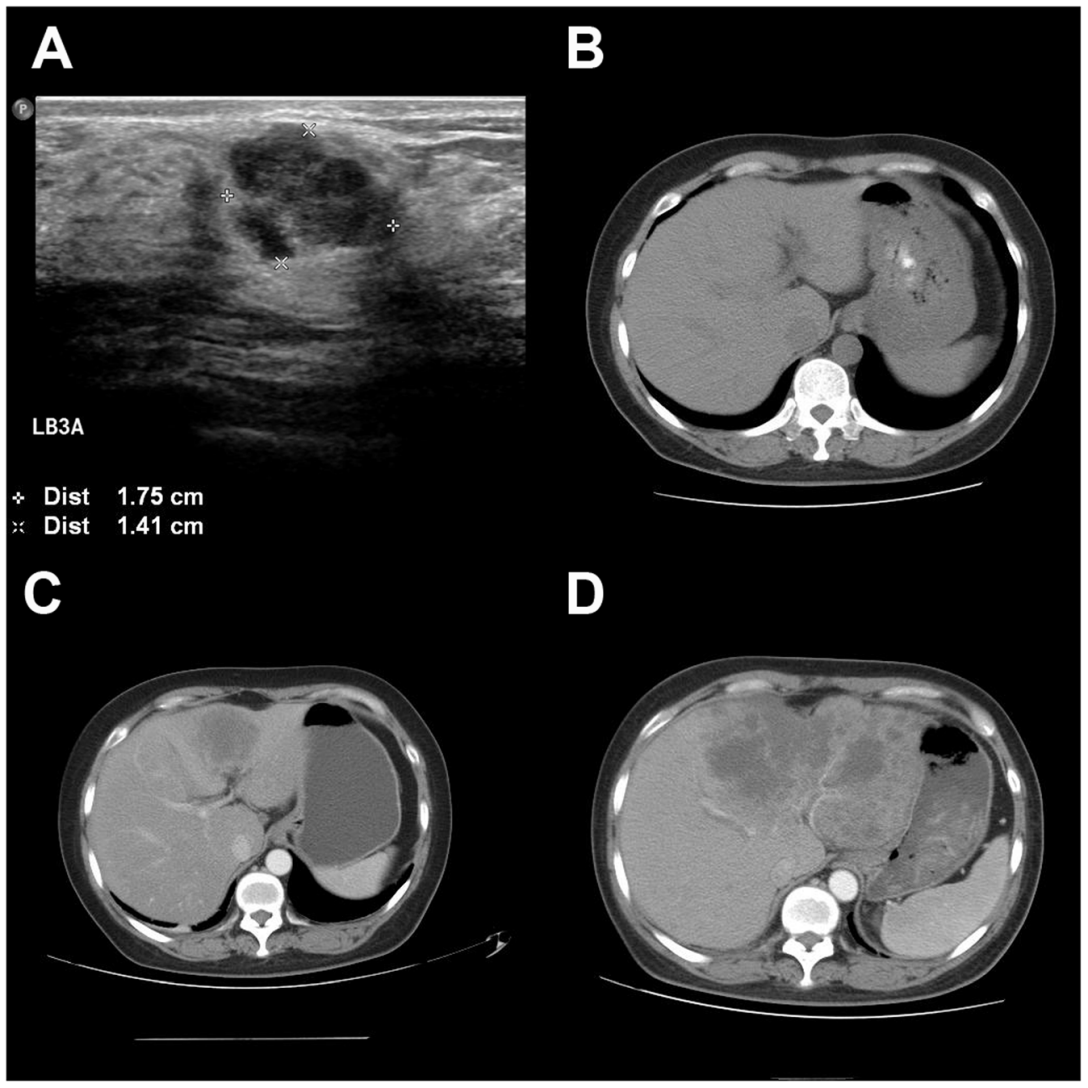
Representitive images of one patients with PR negative breast cancer. (A) Ultrasonography of the primary breast tumor before surgery (Dec, 2009). (B) No metastatic lesion showed on abdominal CT scan before surgery(Dec, 2009). (C) Liver metastasis confirmed by core needle biopsy 10 months after the surgery (Oct, 2010). (D) Last abdominal CT scan shortly before the patient died, which suggested no response to chemotherapy (Sept, 2011).
